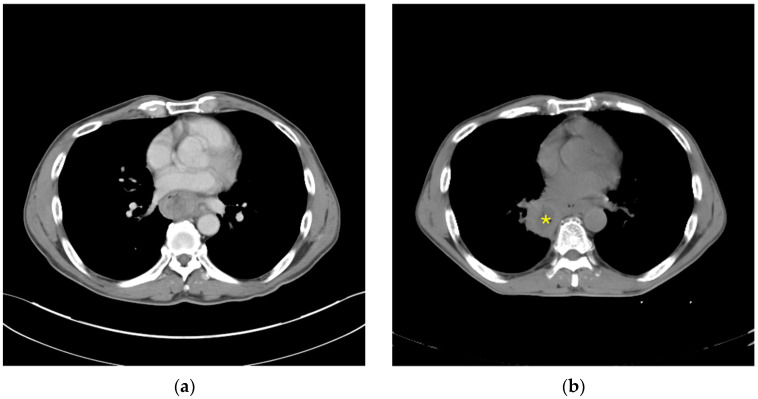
(a) Initial computed tomography showing an irregular enhancing tumor in the lower thoracic esophagus without definite invasion of adjacent organs.; (b) Computed tomography showing a right lower lobe abscess (*) adjacent to the esophageal tumor, supporting the clinical suspicion of esophageal perforation and esophagopulmonary fistula.
